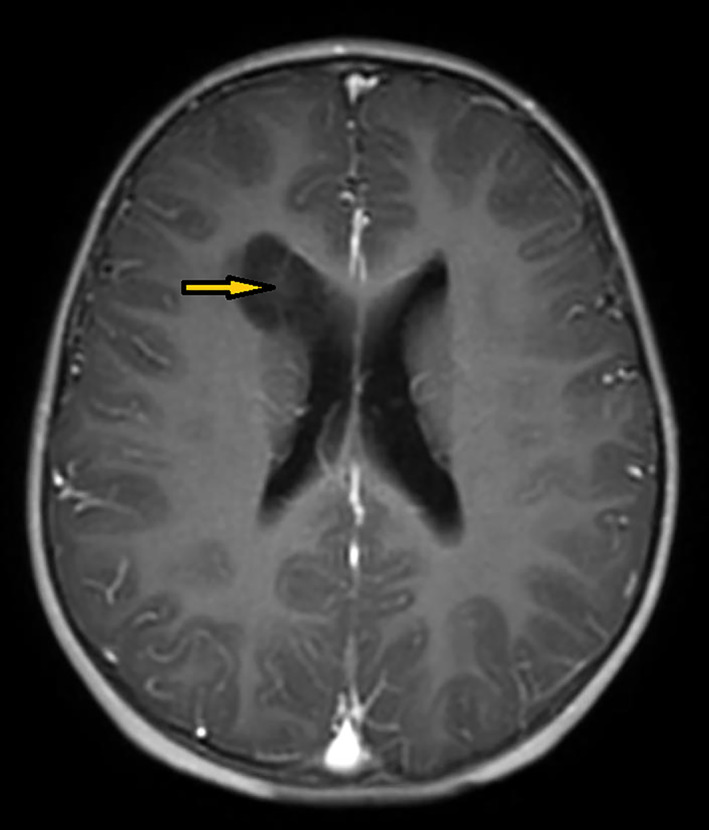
Axial T1‐weighted contrast enhanced magnetic resonance imaging shows the low intensity mass was located on the front edge of the right brain ventricle, with a few darker strands and no enhancement (arrowhead).
